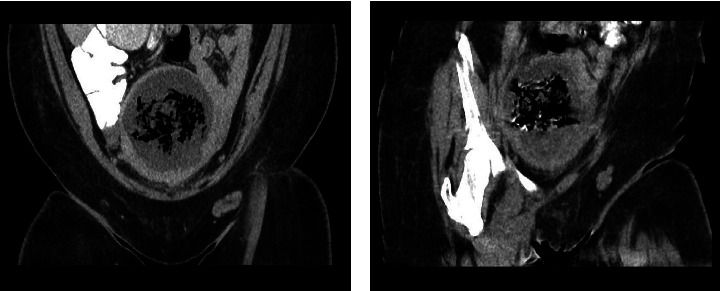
CT scan of the pelvis (a) and (b) showing coronal views of the uterus with areas of necrosis.
